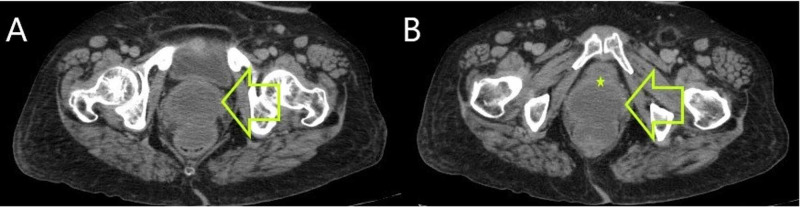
(A and B) Pelvic CT scan showing a mass of the anterior rectal wall (yellow arrows) with an invasion of the posterior vaginal wall (yellow star)
